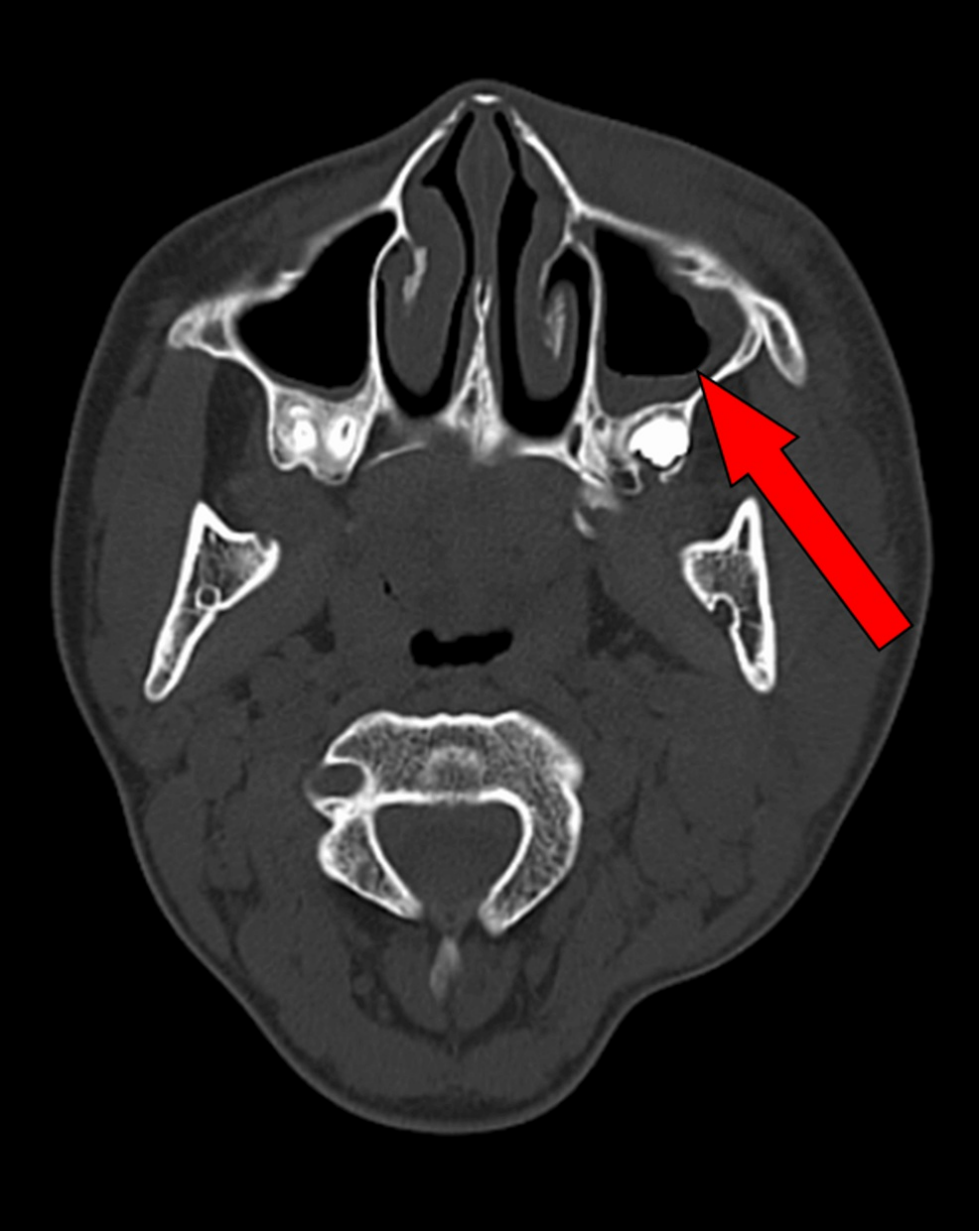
The axial section of the computed tomography scan showing the maxillary sinus. The arrow shows the thickening of the maxillary sinus walls on the left side.
